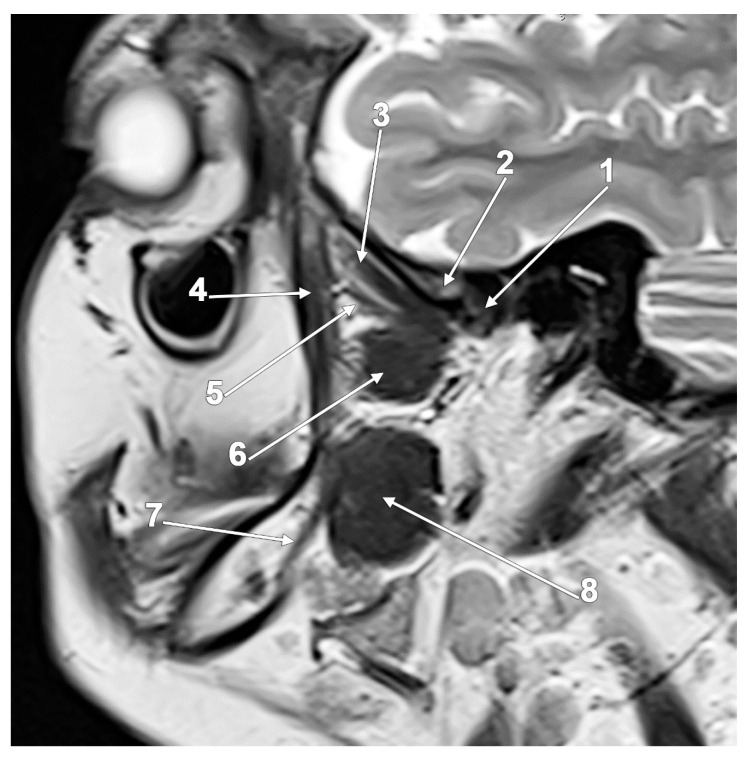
Sagittal MRI slice through the lateral pterygoid muscle (LPM), where three heads/bundles are found: 1. head of the mandible; 2. articular tubercle; 3. superior head of the LPM; 4. deep fibers of the temporal muscle; 5. middle head of the LPM; 6. inferior head of the LPM; 7. mandibular canal; and 8. medial pterygoid muscle.
